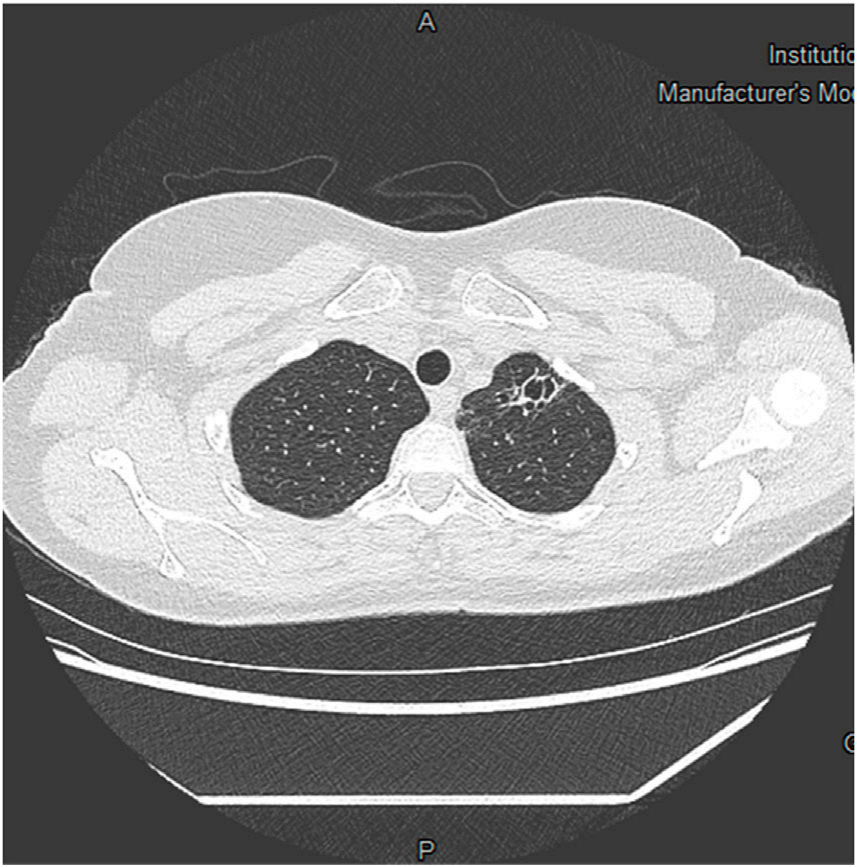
Chest CT scan showing apical bronchiectasis.
